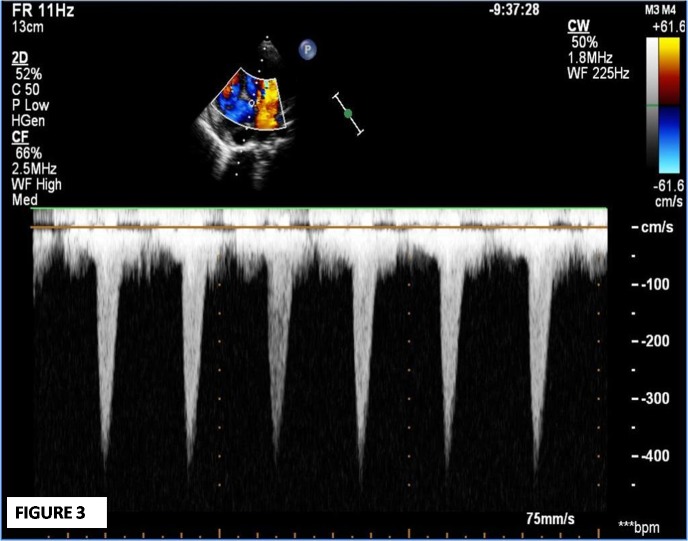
Figure 3:Continuous wave (CW) doppler interrogates the flow from left ventricular cavity toaneurysmal cavity during systole.
